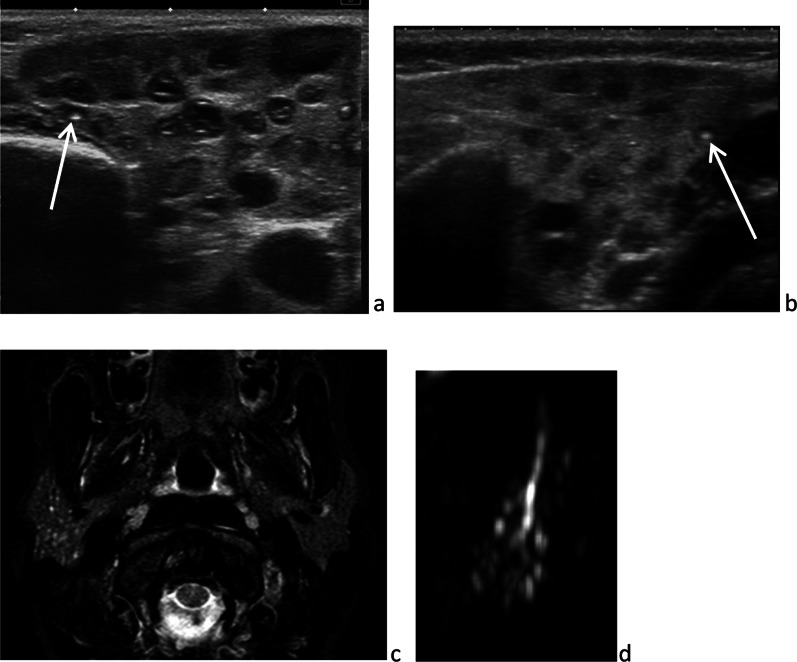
Juvenile recurrent parotitis. Multiple foci of salivary secretions scattered in a normal sized gland, with some central calcification [white arrow] and without Stensen’s duct dilatation, on ultrasonography (a and b), axial fat sat *T*_2_-weighted image (c) and MR-sialography (d)
